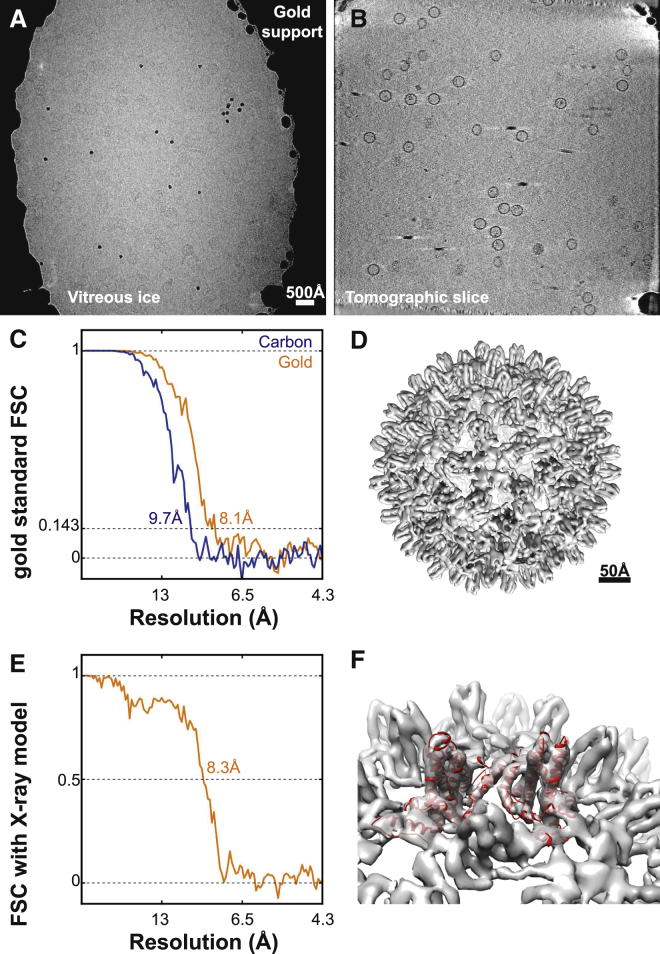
Sub-tomogram Averaging with Reduced Radiation-Induced Motion (A) Representative image from a tilt series of the hepatitis B capsid sample imaged on a gold support grid. The support is seen as a high-contrast feature. (B) Slice from a tomogram reconstructed from the tilt series data. The scale bar applies to (A) and (B). (C) FSC curves showing the estimated resolution from the refinements of data from carbon grids and gold supports. (1,145 HBV capsid particles were used in both refinements; for the gold supports these came from 11 tomograms.) (D) Isosurface representation of the output volume from the refinement with data collected on gold supports. (E) FSC curve of the sub-tomogram averaging model from the refinement with data collected on gold supports against the X-ray structure. (F) The X-ray structure is shown fitted into the sub-tomogram averaging map where secondary structure elements are clearly resolved. See also Figures S3 and S4.
